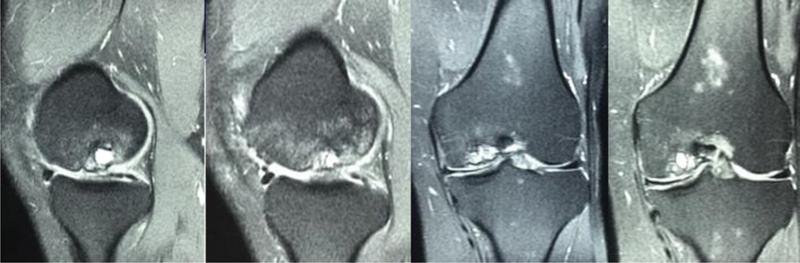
Coronal and sagittal magnetic resonance imaging sequences showing failure mosaicplasty integration of the medial femoral condyle and previous partial meniscectomy.
